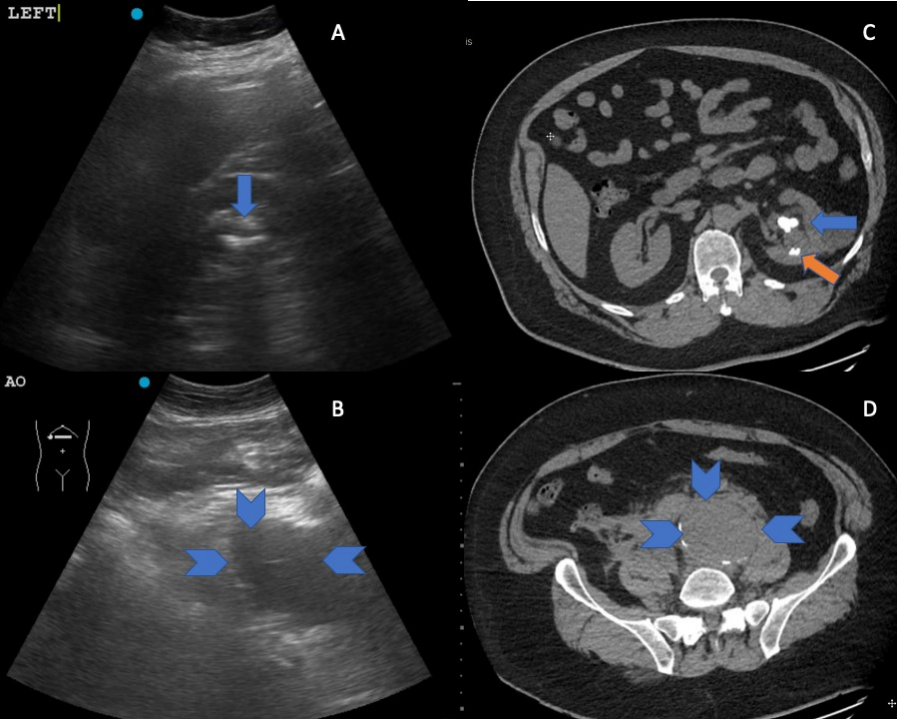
A) POCUS image of left kidney with large stone in the renal pelvis (blue arrow) and hydronephrosis. B) left iliac artery (blue chevrons). C) CT image of left kidney the renal pelvis stone (blue arrow) and lower pole non-obstructing stones (orange arrow) and D) left iliac artery with aneurysm (blue chevrons).
